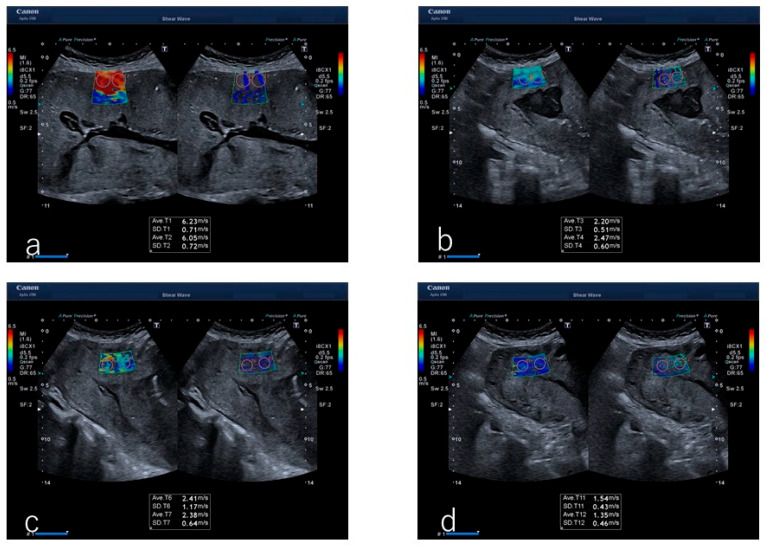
Image of ultrasound shear wave elastography during the third stage of labor in the case of a participant. (a) Immediately after infant delivery, (b) 240 s later, (c) 300 s later, and (d) 360 s after infant delivery.
